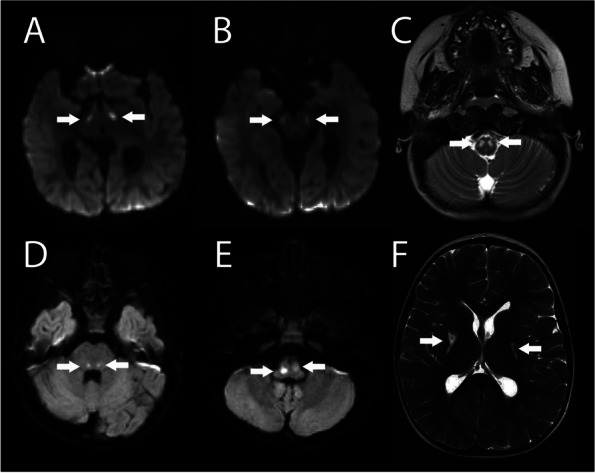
Images from MRI performed at 15 month (A-C) and at 46 month of age (D-F). The white arrows point to the key findings in each case. A-B are diffusion weighed images (DWI) representing acute infarcts in the sub-thalamic nuclei and brainstem. C is a T2-weighted (T2w) image demonstrating similar changes in the medulla and upper cord. D-E are DWI representing acute infarcts in the posterior pons and medulla. The findings are asymmetric in (E). F is a T2w image demonstrating the chronic infarcts in the basal ganglia
